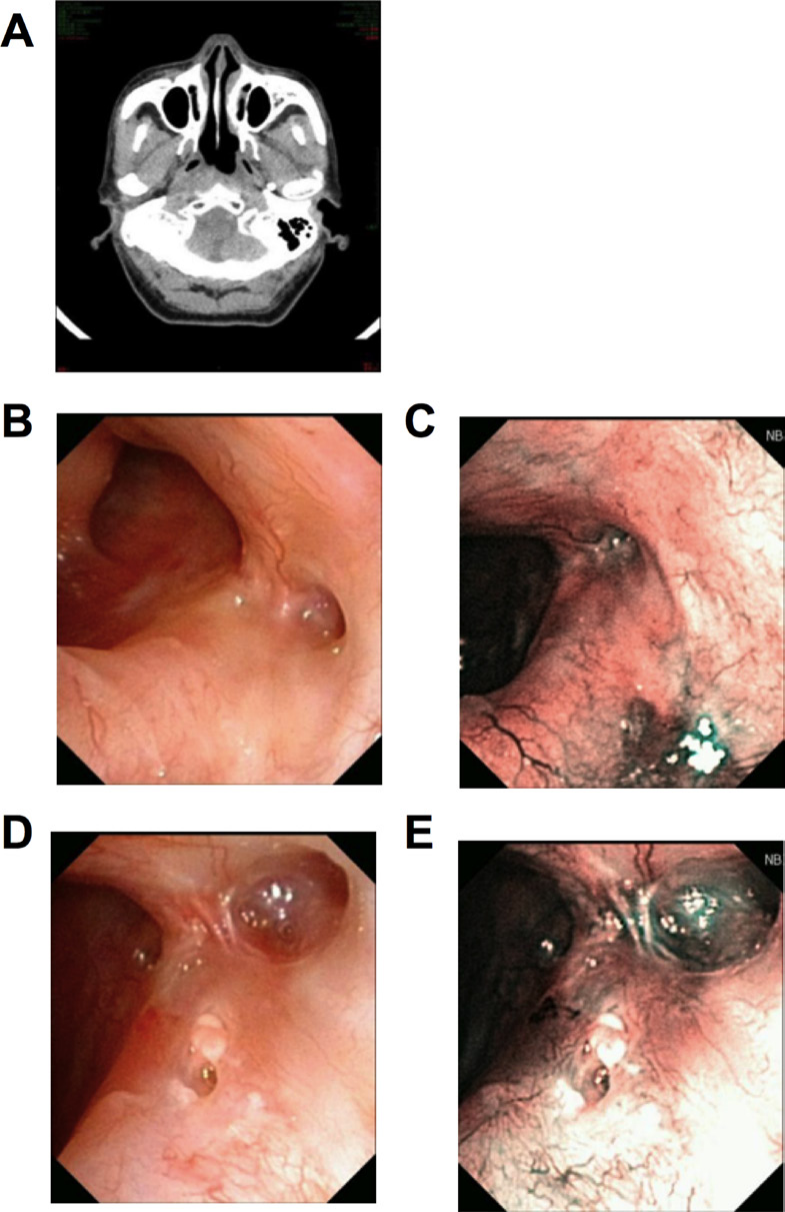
Recurrent patient of NPC CT scan showed swollen longus capitis and parapharyngeal space invasion on the right side (A). Under WL, after radiotherapy, nasopharyngeal tissue had local adhesion. Mucosa slight bulging, but smooth, the color and lustre was normal (B and C). Under NBI, there was no positive found as brownish spots (D). The CNB puncture point 1 month later, under WL and NBI respectively (E and F).
